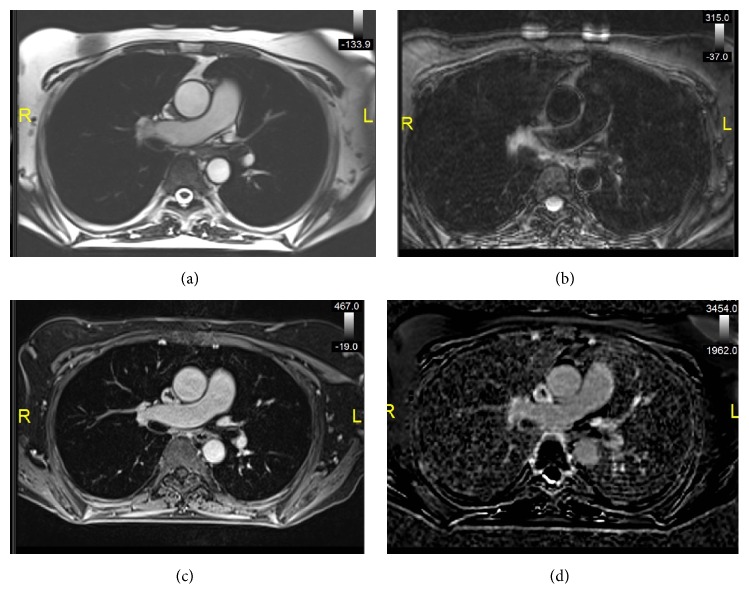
Cardiac magnetic resonance evaluation. (a) Axial SSFP image shows a hypointense structure conforming to the medial lower SVC wall. (b) Axial T2 SPAIR MR image shows isointense T2 signal within the structure. (c) Axial T1 postcontrast image demonstrates a well-defined intravascular filling defect along the SVC wall. (d) Late gadolinium enhancement phase sensitive inversion recovery image demonstrates hypoenhancement of the intravascular structure. Findings are compatible with bland thrombus.
